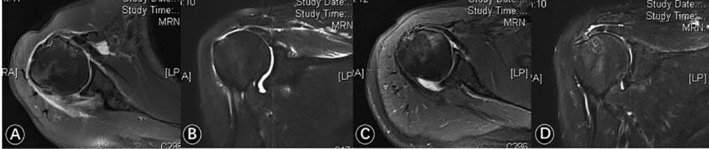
(A) Preoperative MRI showing subscapularis tears and long head of the biceps (LHB) tendon injury; (B) concomitant supraspinatus tears; (C) 6 months postoperatively, MRI showed that the subscapularis tendon healed well; and (D) MRI showed that the supraspinatus tendon healed well 6 months after surgery.
